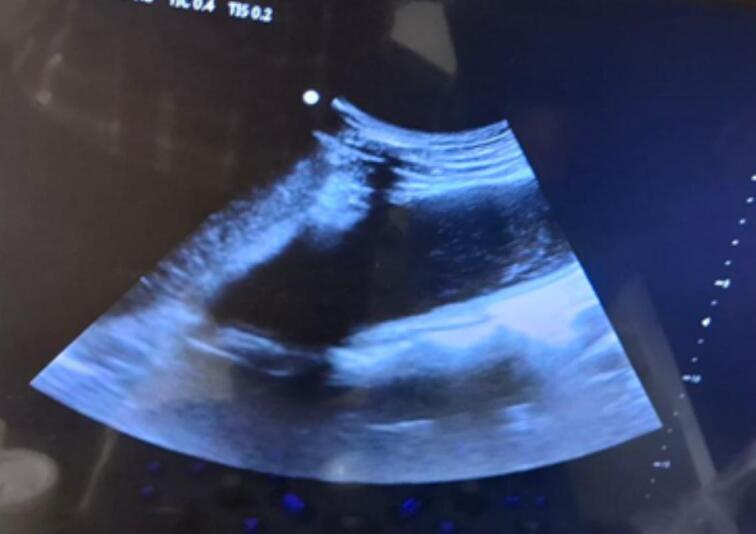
Abdomino-pelvic ultrasound showed 18 cm × 6 cm × 5 cm measuring echo complex left retroperitoneal collection on the left psoas muscle.
